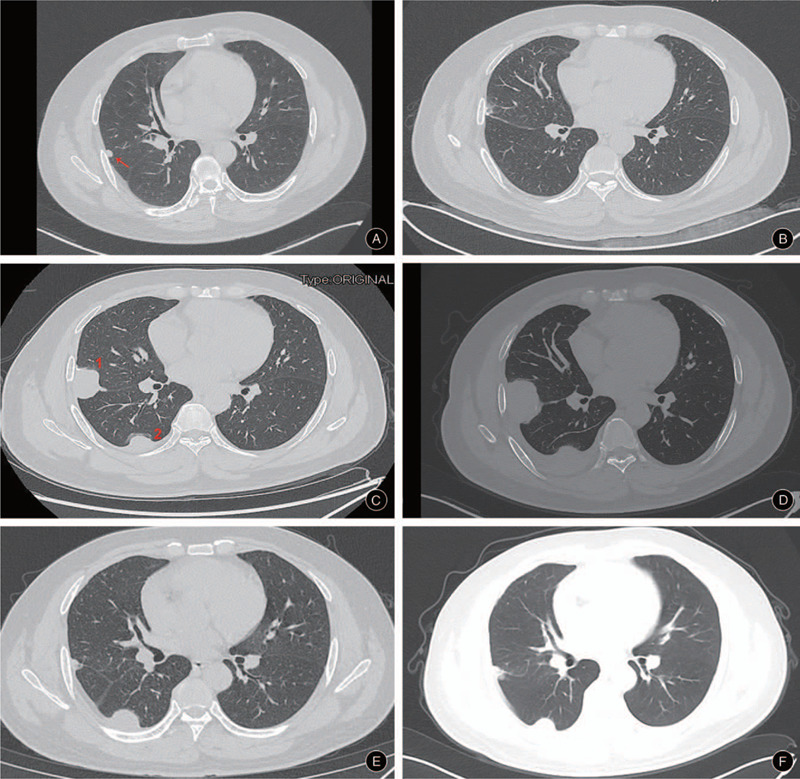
(A) The pulmonary lesion in the right upper lode scanned through CT on July 1, 2018; (B) The right upper lode after the thoracoscopic pulmonary nodule resection (August 15, 2018); (C) The pulmonary lesion relapsed 4 months after the pulmonary nodule resection (November 23,2018) and lesion 1 and 2 were 38.8 mm × 24.8 mm and 14.2 mm × 9.2 mm in size, respectively; (D) The pulmonary lesion enlarged 1 month after the initial administration of Anlotinib (December 22, 2018) and lesion 1 and 2 were 49.3 mm × 34.2 mm and 37.0 mm × 20.2 mm in size, respectively; (E) The pulmonary lesion decreased 5 month after the initial administration of Camrelizumab (June 2, 2019) and lesion 1 and 2 were 8.4 mm × 8.0 mm and 16.6 mm × 13.4 mm in size, respectively; (F) The pulmonary lesion scanned 12 month after the initial administration of Camrelizumab (January 2, 2020) and lesion 1 and 2 were 12.4 mm × 9.0 mm and 16.5 mm × 13.2 mm in size, respectively.
